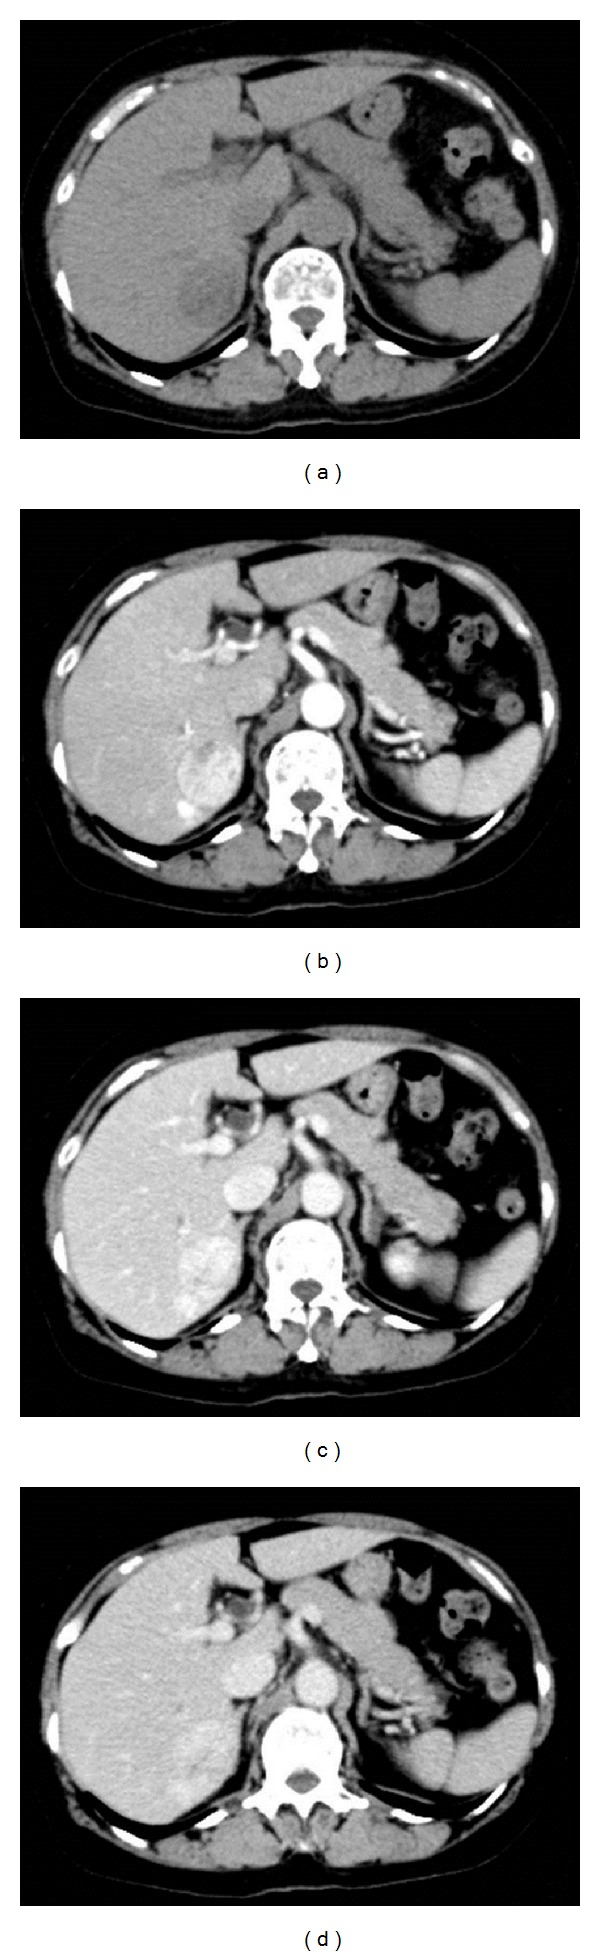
Dynamic contrast enhancement computed tomography (CT). Plain CT revealed a low-density tumor in segment 6 (a). Tumor hypervascularity in the arterial phase (b). Hypervascular lesions remained in the portal phase (c) and late phase (d).
